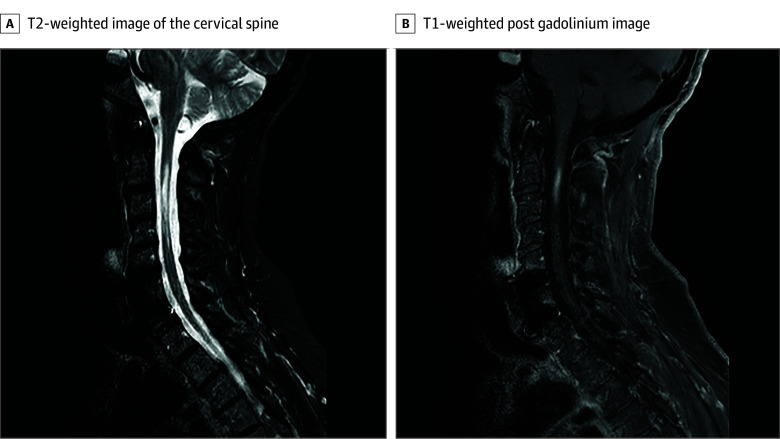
Magnetic Resonance Imaging (MRI) of Active Myelitis in Cervical Spine The MRI images show active myelitis at the C2 to C5 levels on the T2-weighted image of the cervical spine (A) and the T1-weighted post gadolinium image (B).
